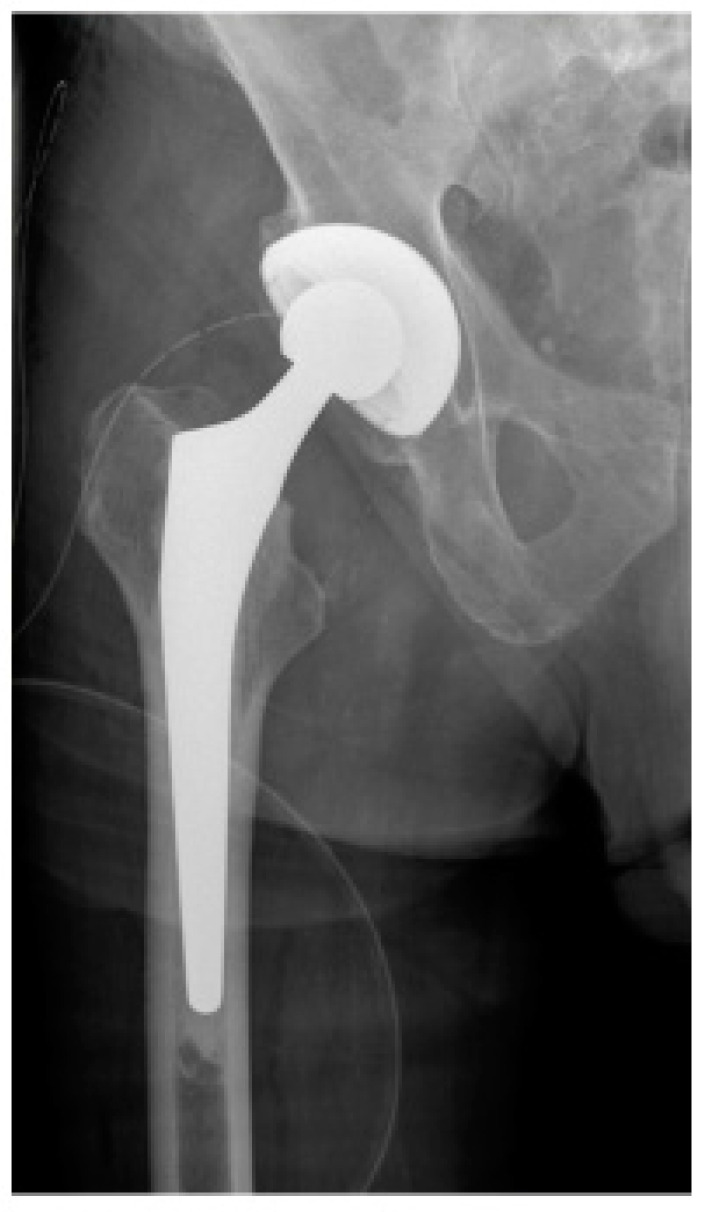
Early postoperative X-ray of the right hip joint in AP view of a male patient after femoral neck fracture who underwent total hip arthroplasty (THA).
